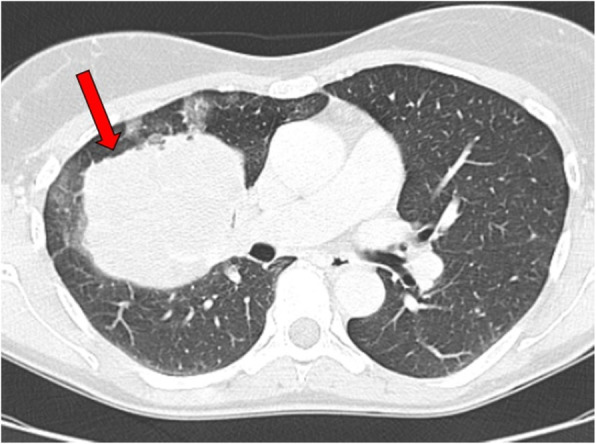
CT scan of chest performed in October 2014 because of the persistence of chronic cough, showing a mass of 90 mm in the right upper lobe (case report 1)
